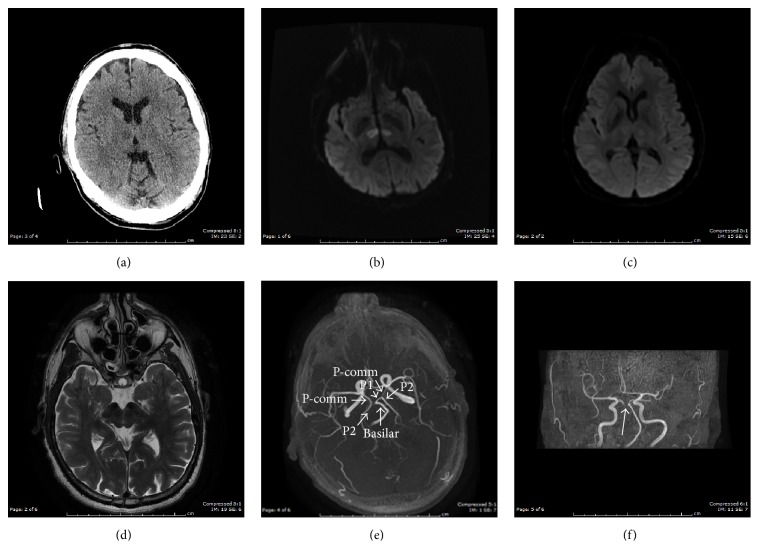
(a) CT head on July 16, 2015, in ER showed bilateral thalamic hypodensities which may represent infarcts; (b) MRI (DWI) on July 16, 2015, showed acute bilateral medial thalamic infarcts, consistent with artery of Percheron territory; (c) MRI (DWI) after two weeks of MICU management on July 31, 2015, showed improved; (d) T2-weighted MRI showed no midbrain involved; (e, f) MRA showed occluded or atretic right P1 segment. The right PCA is patent and supplied by the P-comm. The left PCA is patent.
